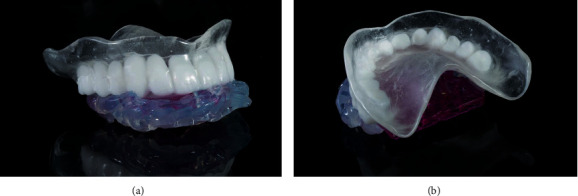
(a) Frontal view of the radiographic template. (b) View on the base area of the radiographic template.
